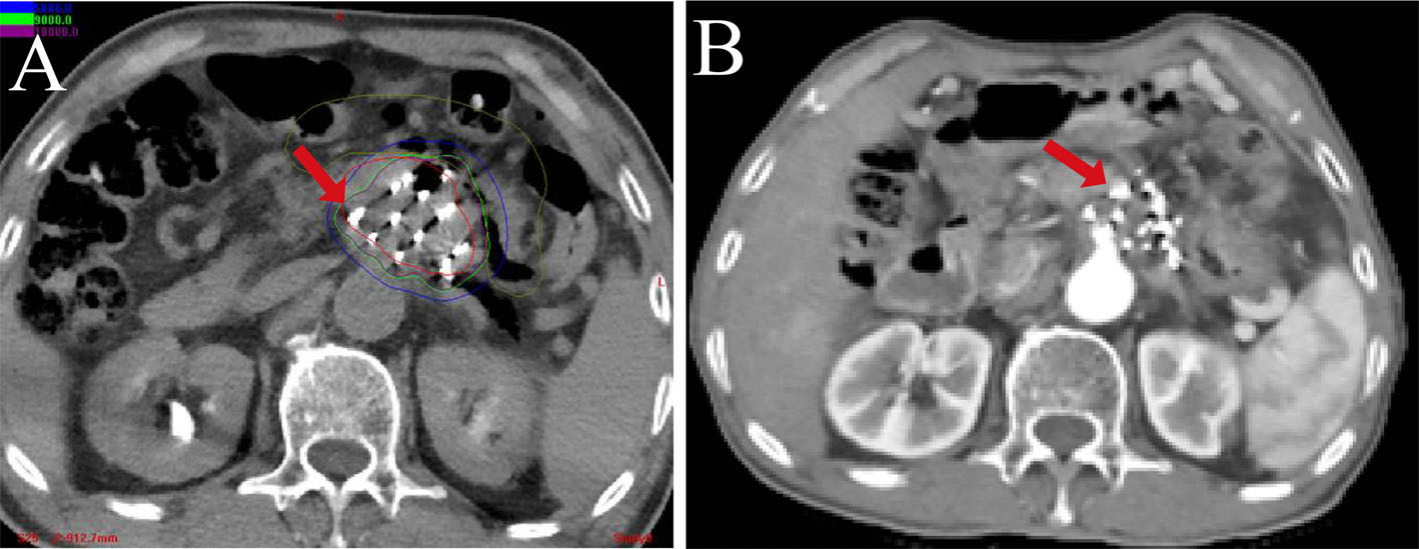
The CT-scan image of 125I-RSI BT in pancreatic head cancer. (A) The postoperative actual dose distribution. (B) The postoperative follow-up at 6 months.
